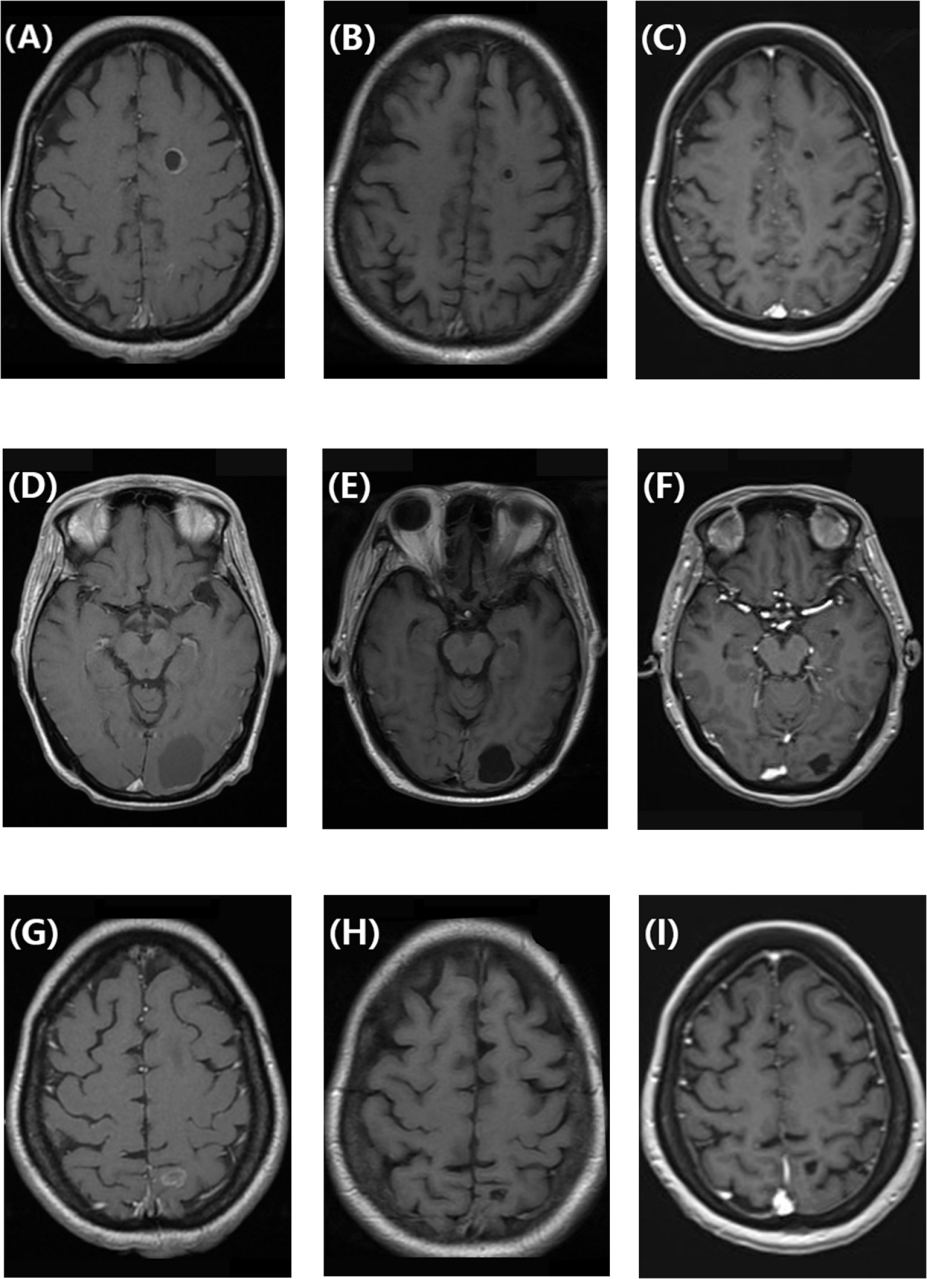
(A, D, G) Enhanced T1-weighted brain magnetic resonance image showing cystic brain metastases in a patient with hormone receptor-positive/human epidermal growth factor receptor 2-negative breast cancer before treatment with abemaciclib and fulvestrant. (B, E, H) Enhanced T1-weighted magnetic resonance image showing that the cystic brain metastases decreased in size after 3 months of treatment with abemaciclib and fulvestrant. (C, F, I) Enhanced T1-weighted magnetic resonance image showing that the cystic brain metastases remained in partial remission after 9 months of treatment with abemaciclib and fulvestrant.
